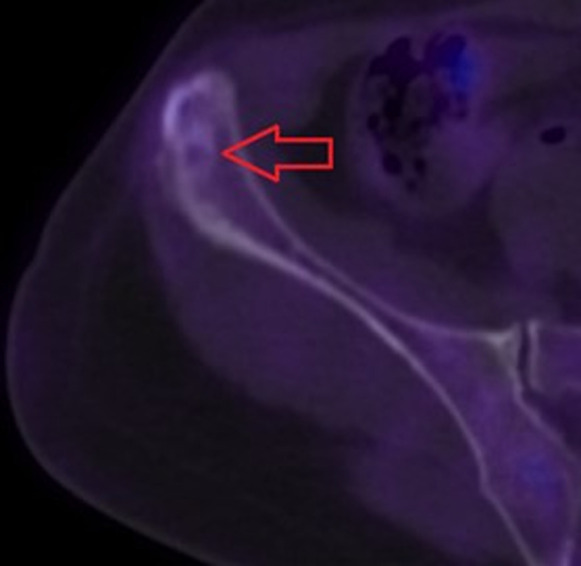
persistent bone metastases on the right pelvic bone detected using bone PET-CT
